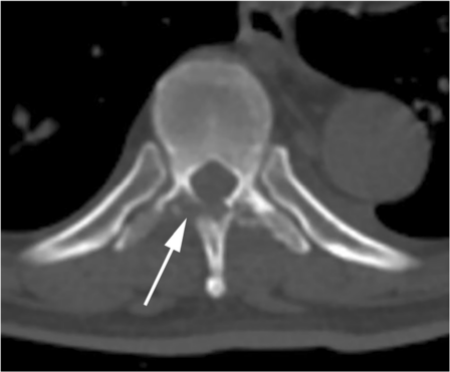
Computed tomography scan of the thoracic vertebra. A destructive focus (single-headed arrow) in the bilateral vertebral plates and transverse processes was detected
